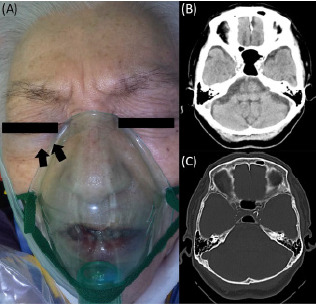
Facial appearance and cranial computed tomography (CT) performed on arrival. Mild periorbital ecchymoses was visible around the right eye (arrows) (A), and cranial CT shows no signs of cranial injury, skull fracture, or a carotid-cavernous fistula (B, C).
